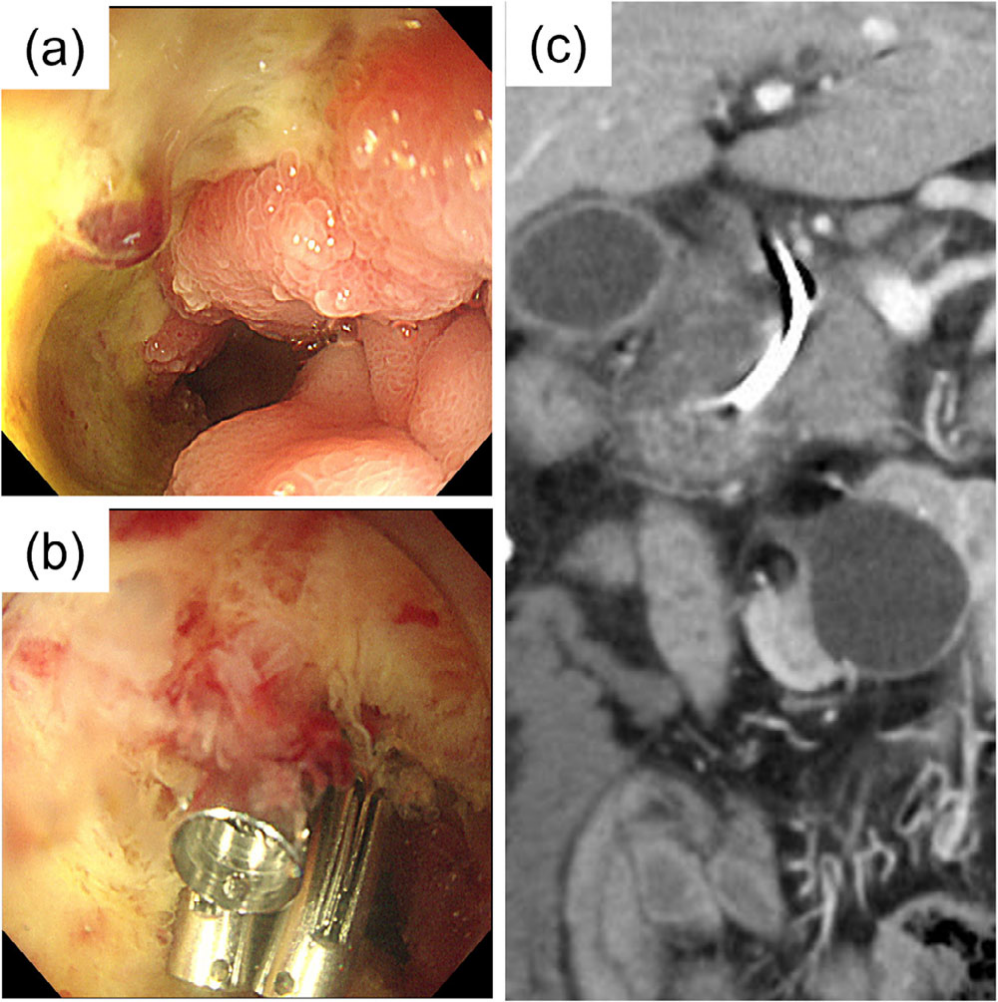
Endoscopic and computed tomography images of the duodenum showing a hemorrhagic event. (a) Post‐bulbar duodenal ulcer with an exposed vessel. (b) Endoscopic hemostat with three hemoclips (SureClip; Micro‐Tech Co., Ltd). (c) Thickened wall in the second part of the duodenum with the biliary stent. The presence of a thickened wall with internal heterogeneity was also misleading.
